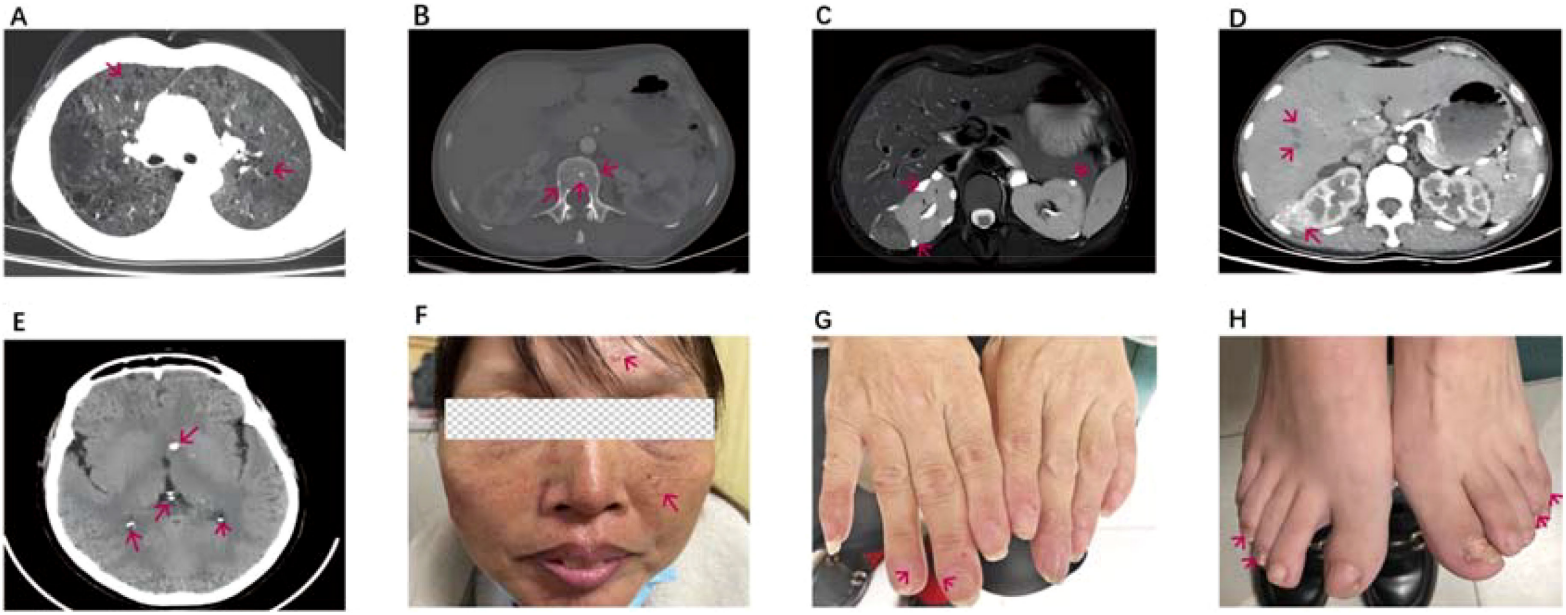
(A) CT scan of the chest shows sac -like translucent and ground-glass opacities in both lungs (B) CT of the spine shows multiple high-density nodules in the vertebrae. (C) MRI enhancement shows abnormal enhancement of multiple nodules in the liver and bilateral kidneys. (D) CT enhancement showed very low-density opacities in the liver and kidney, as well as intrarenal mass opacities. (E) CT brain shows bilateral paraventricular nodular hyperdensity. (F) a few hemangiomatous rash on the patient's face and fibrous plaques on the forehead. (G) Fibroids on the fingers (H) Fibroids on the toes. The red arrows indicate the abnormalities observed on imaging and the patient's abnormal signs.
